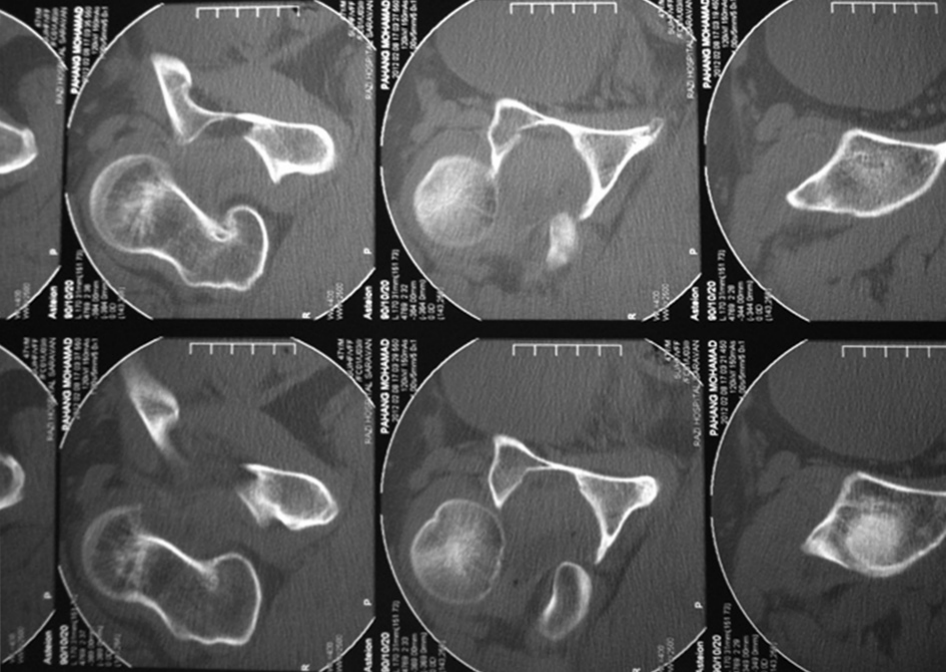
Computed Tomography of the Right Hip
